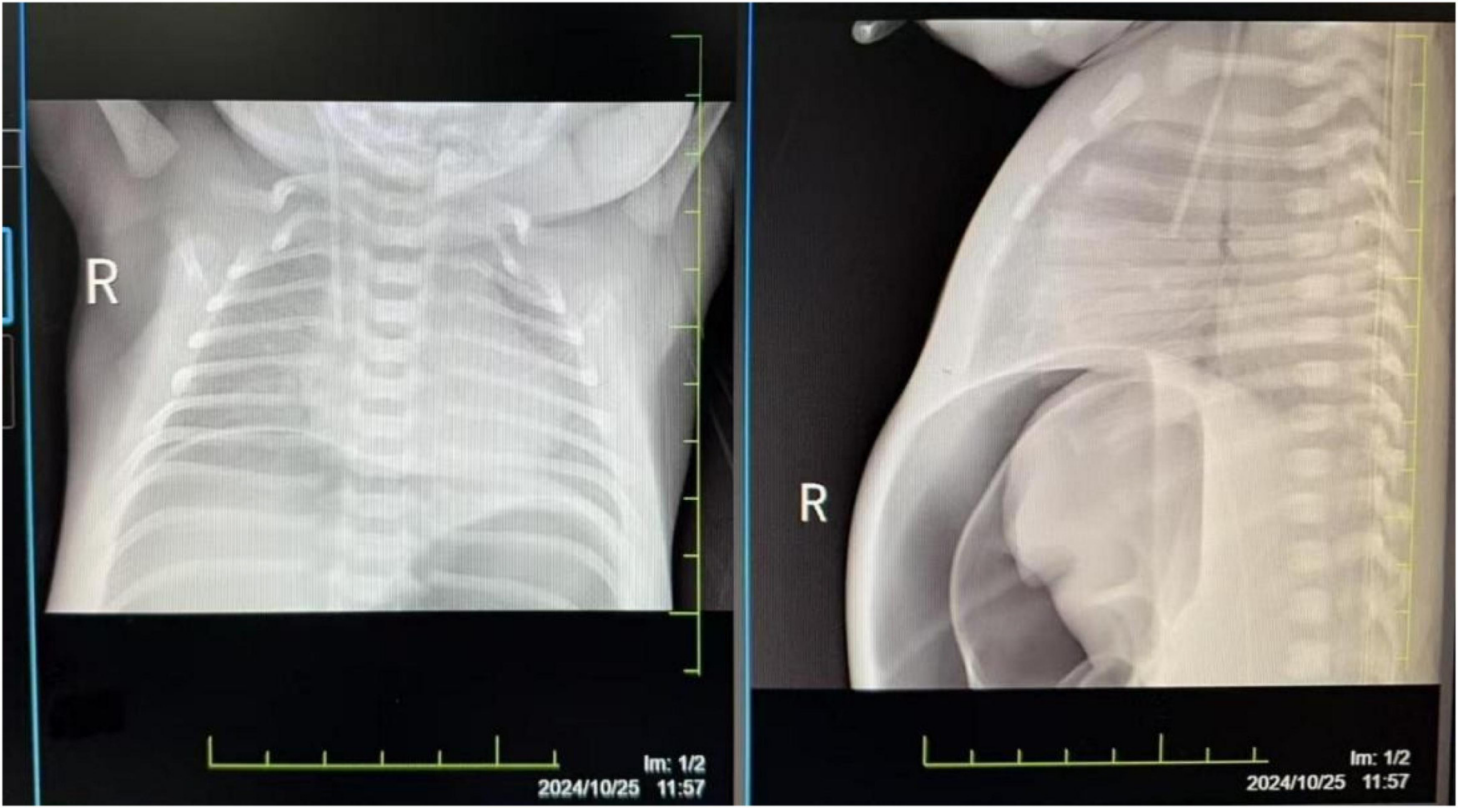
Abdominal radiograph taken on the fourth day after birth, showing pneumoperitoneum.
